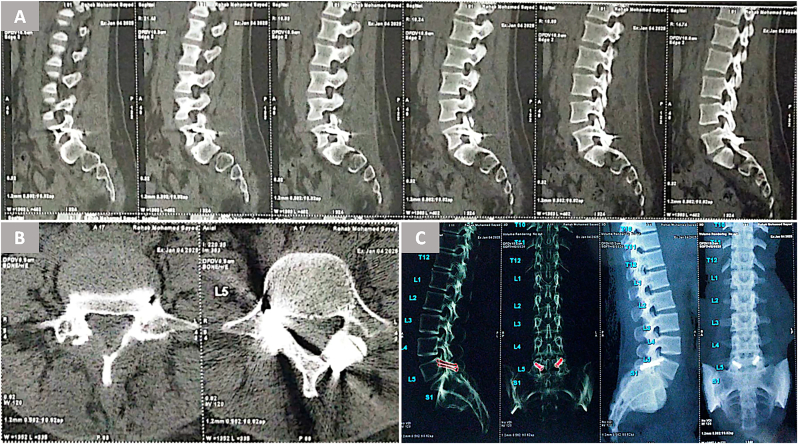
Postoperative CT at 18 months (same patient as Fig. 3) demonstrating complete bony fusion via bridging trabecular bone across the pars defect on sagittal (A), axial (B), and 3D (C) reconstructions.
